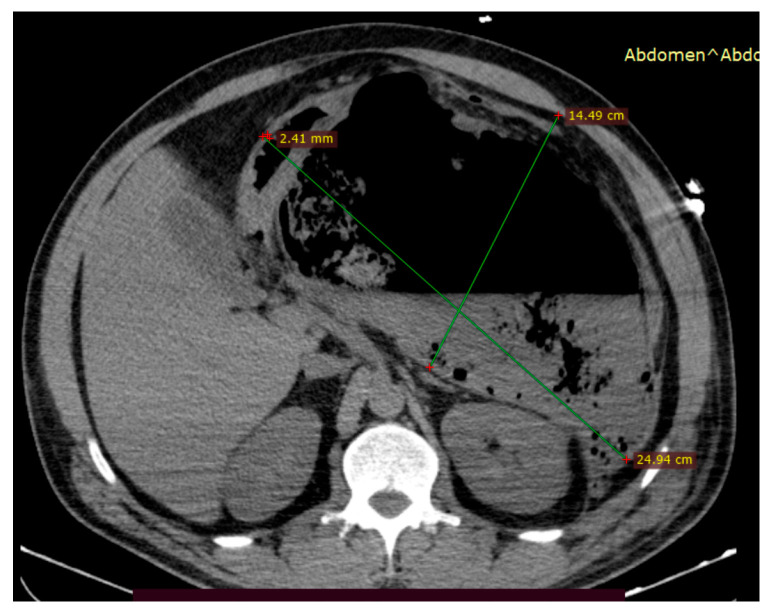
An abdominal CT scan performed within 24 h of admission showed a large mixed-type partially encapsulated peripancreatic necrotic collection (approximately 50% necrosis) causing organ compression and severe abdominal compartment syndrome.
